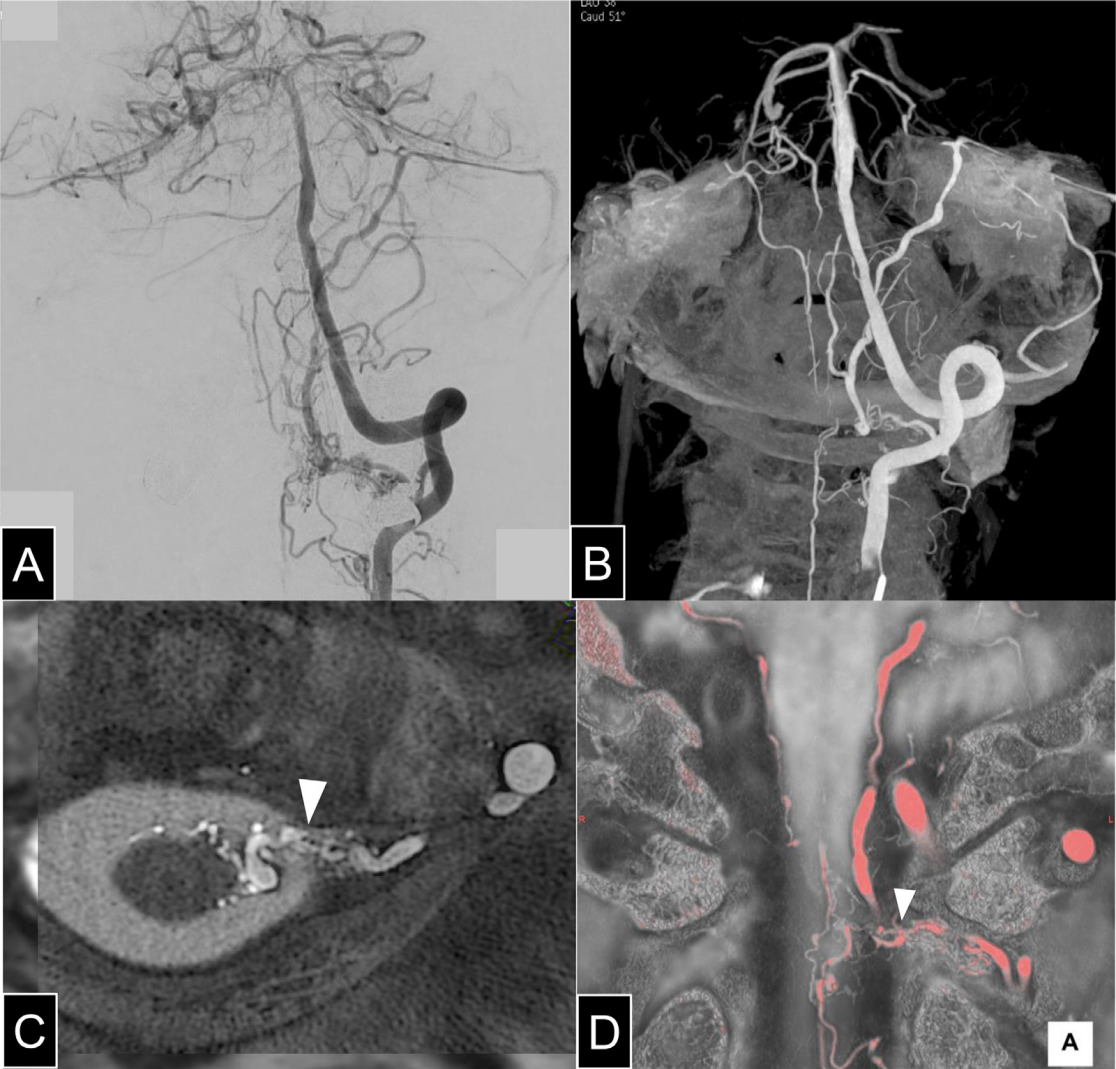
(A) An anterior-posterior view of left vertebral angiography demonstrates a radiculomeningeal artery arising from the V3 segment and extending medially, forming an arteriovenous fistula with venous drainage observed both superiorly and inferiorly. Image quality in the surrounding area was partially degraded due to digital subtraction artifacts caused by a dental metal crown. (B) 3D rotational angiography of the left vertebral artery demonstrates a radiculomeningeal artery (white arrow) forming a fistulous connection with the anterior lateral spinal vein, with clear visualization of the venous drainage routes. Bilateral lateral medullary veins and lateral pontine veins are visualized, with marked dilation observed on the left side. The venous drainage proceeds via the superior petrosal sinus and petrosal vein into the basal vein of Rosenthal. (C) Axial fused image of heavily T2-weighted MRI and 3D rotational angiography of the left vertebral artery. The white arrowhead indicates the shunt point. Based on the vessel caliber change observed on the dura mater, the lesion was diagnosed as a dural arteriovenous fistula (DAVF). (D) Coronal fused image of heavily T2-weighted MRI and 3D rotational angiography of the left vertebral artery, with vascular structures rendered in red. The white arrowhead indicates the shunt point located on the dura mater.
